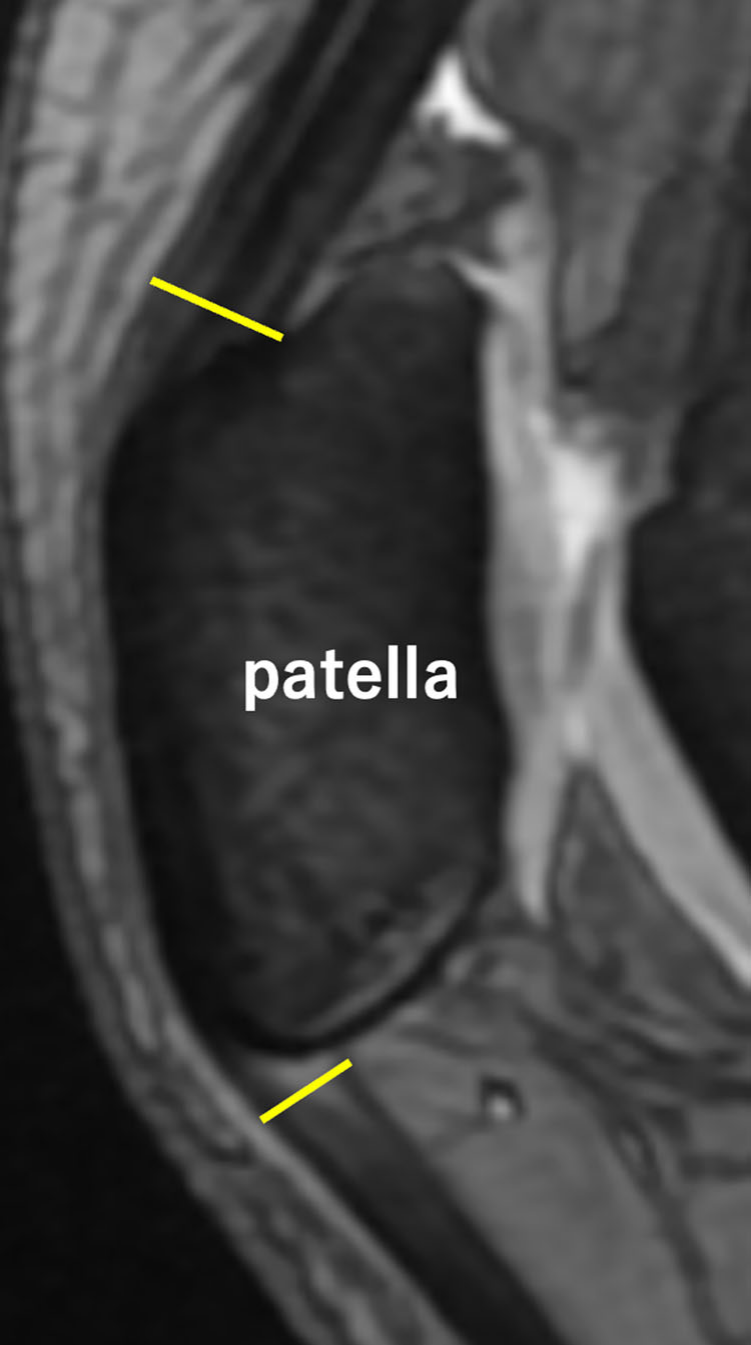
Measurement of tendon thickness. Sagittal magnetic resonance imaging showing the measurement of quadriceps tendon and patellar tendon thicknesses at potential anterior cruciate ligament graft harvest sites. The yellow lines indicate the thickness measurement locations of the quadriceps tendon (upper line) and patellar tendon (lower line). These were measured perpendicular to the tendon at their patellar attachments.
